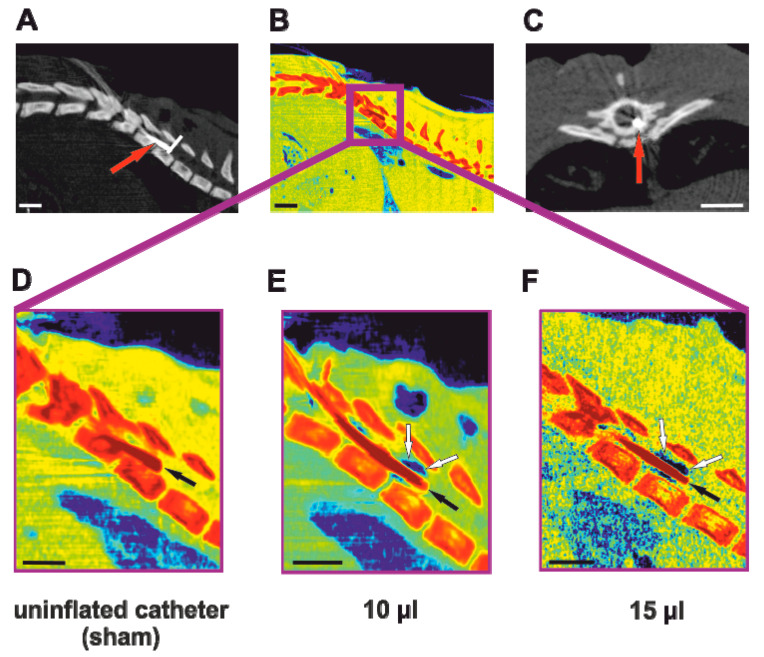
Micro computed tomography (CT) images of ventral epidural catheter placement. In order to evaluate the position of the catheter (red arrows) in front of the spinal cord, micro CT with bone window (A,C) and soft tissue window (B) were applied. Furthermore, detailed images of the deflated balloon (D), as well as inflated to 10 µL (E) and 15 µL (F) volume balloon were obtained. Black arrows show the wire of the catheter, whereas white arrows point to the imaged saline solution indicating the amount of inflated Fogarty´s balloon. Scale bar: 2 mm.
